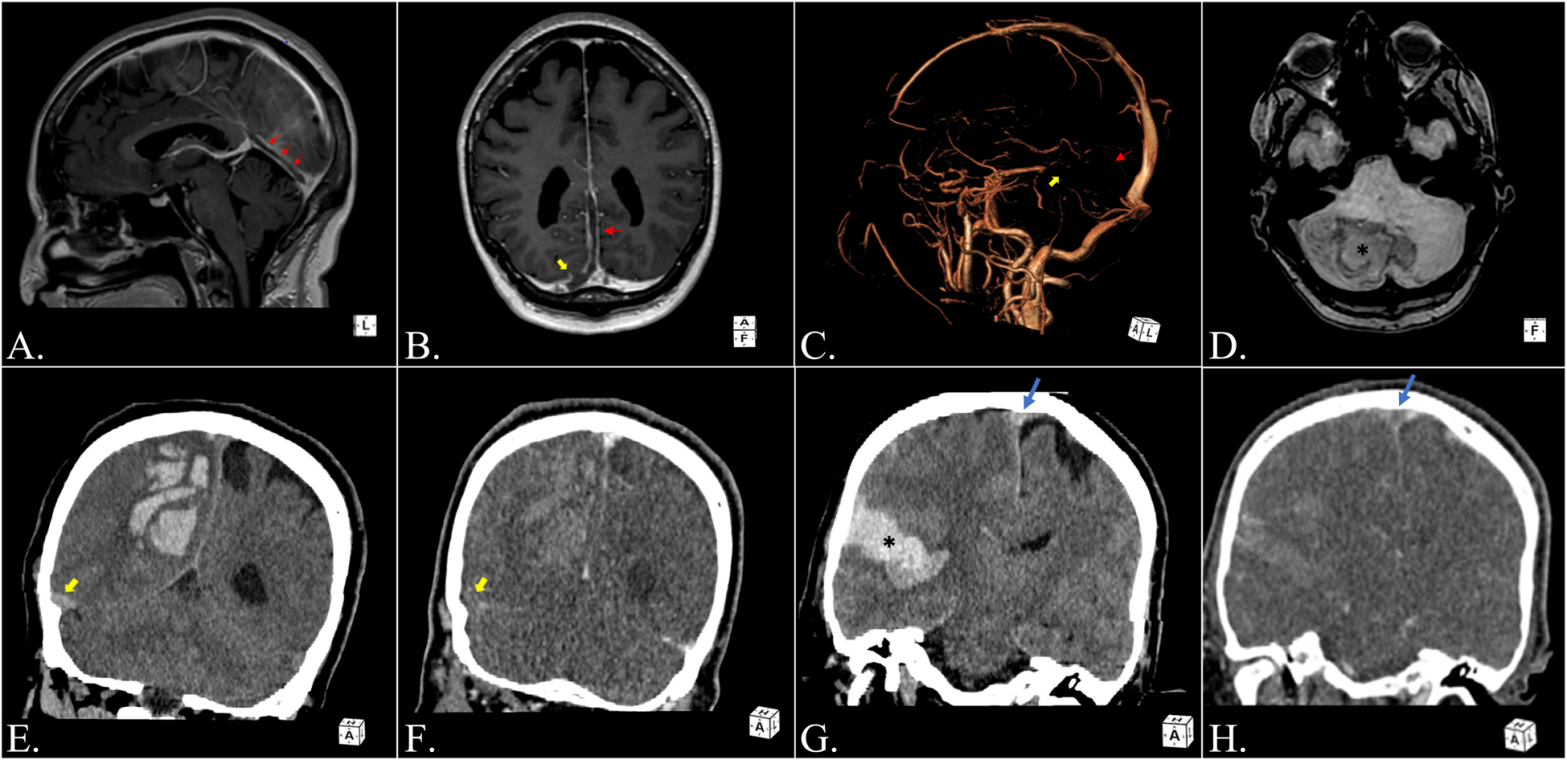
Neuroradiological findings. Patient 1: A, D magnetic resonance imaging (MRI) examination. A, B Sagittal and coronal contrast-enhanced T1-weigthed images depict SS (red arrows) and right TS (yellow arrows) extensive thrombosis with filling defect inside the vessels. C Phase-contrast MRI angiography (PCA) with volume rendering technique shows lack of venous flow in SS (red arrow) and right TS (yellow arrow). D Susceptibility-weighted (SWI) follow-up MRI examination shows acute bilateral cerebellar hemisphere hematoma, prevalent on the right side (asterisk). Patient 2: E, H brain computed tomography (CT scan examination) at clinical onset. E Coronal unenhanced CT scan depicts large right parietal hematoma and ipsilateral TS hyperdensity (“Dense clot Sign”), indicated by yellow arrow. F Coronal enhanced CT scan shows lack of opacization of thrombosed right TS (yellow arrow). G Coronal unenhanced CT scan depicts right temporal hematoma (asterisk) and “Dense clot Sign” at SSS (blue arrow). H Coronal enhanced CT scan shows triangular area of enhancement with a relatively low-attenuating center (“Empty delta Sign”) at thrombosed SSS (blue arrow). Straight sinus (SS), superior sagittal sinus (SSS), transverse sinus (TS)
